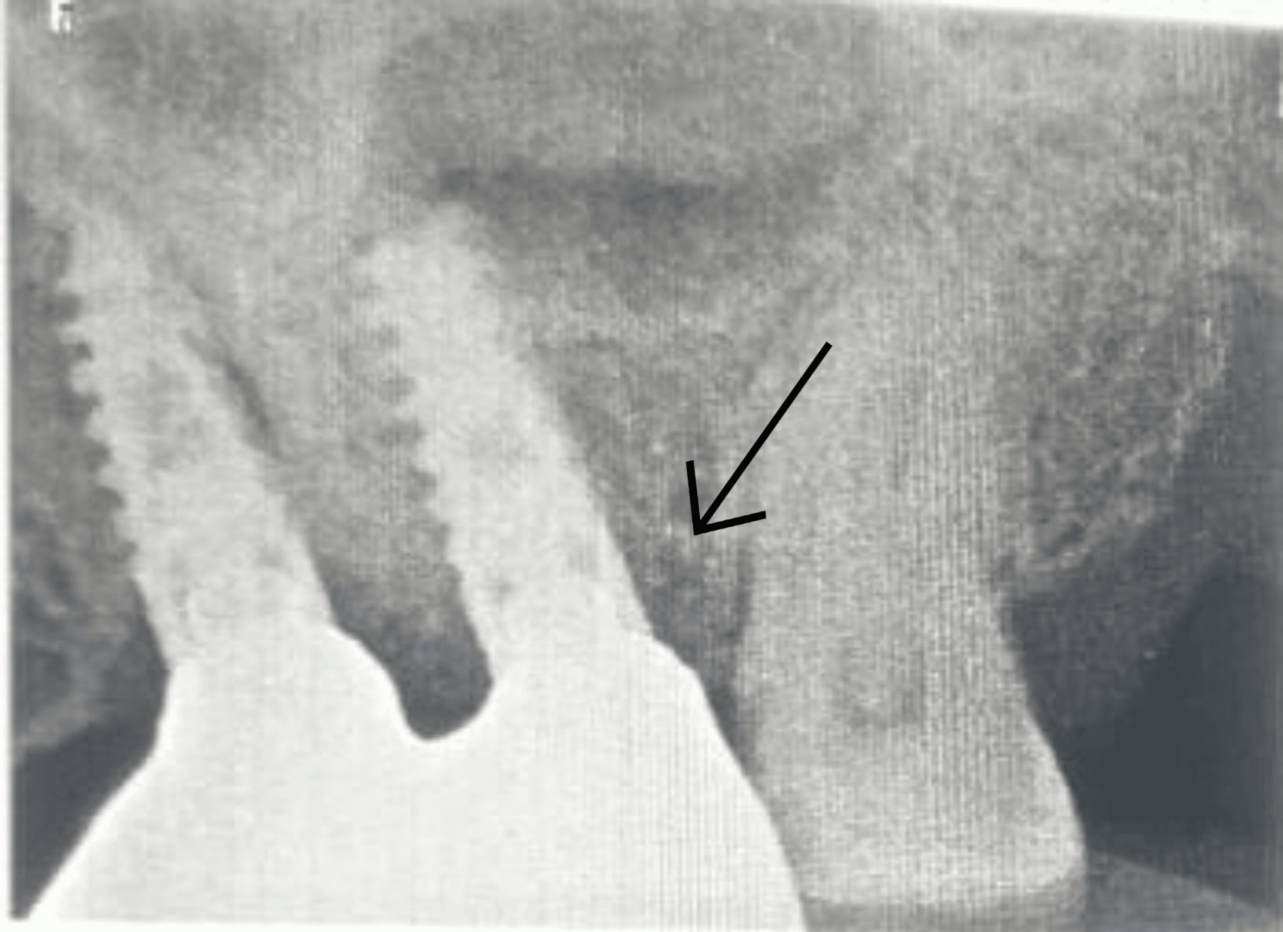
Follow-up IOPA 14 months after prosthetic loading. The arrow indicates the marginal bone stability. IOPA: intraoral periapical radiograph
